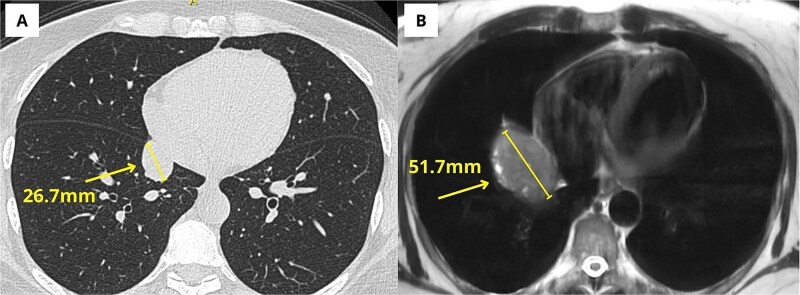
(A) Chest computed tomography from February 2022, showing a right paracardiac lesion reported as a pericardial cyst. (B) Chest MRI from July 2025 showing the same lesion as a well-defined oval mass with clear interval growth.
